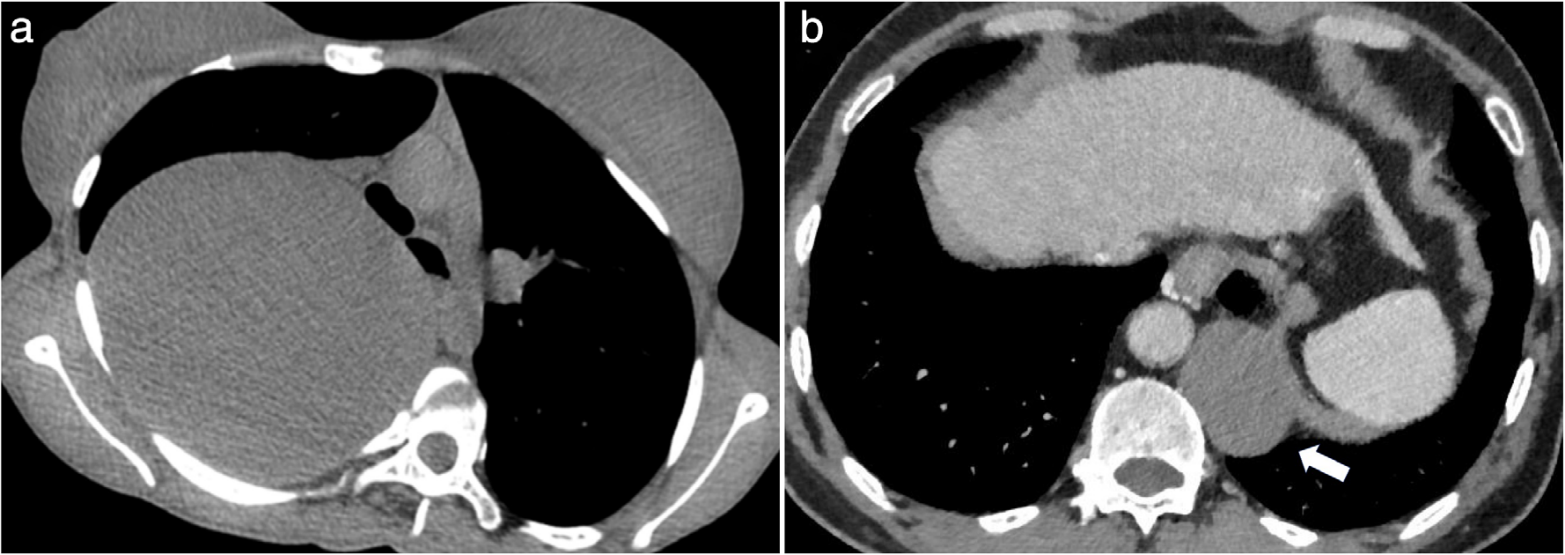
Chest CT showed two primary mediastinal cysts with serious complications caused by the compression of the cysts. (a) A giant mediastinal bronchogenic cyst compressed the right thoracic cavity severely and led to the deformity of the trachea. (b) Compression of the esophagus by an esophageal cyst which led to dysphagia (arrow).
